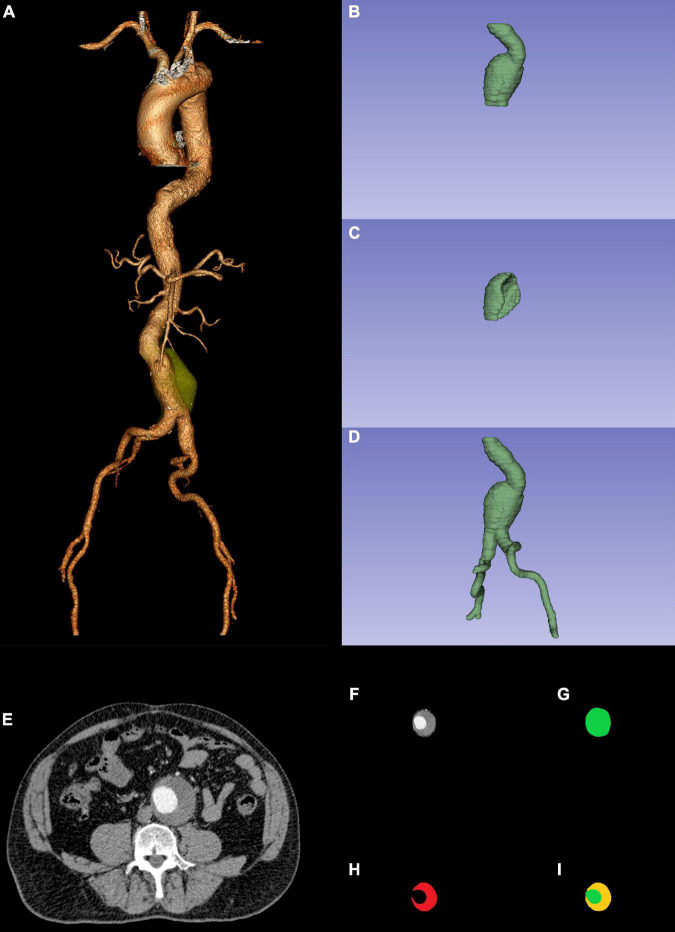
Preoperative CTA reconstruction (A); AAA sac ROI (B); ILT ROI (C); AAA ROI (D); AAA original ROI CTA slice (F) was the combination between AAA ROI CTA slicer (G) and original ROI CTA slice (E); CTA slicer (I) was the combination between AAA sac ROI CTA slicer (G) and ILT ROI CTA slicer (H). CTA, computed tomography angiography; AAA, abdominal aortic aneurysm; ROI, region of interest; ILT, intraluminal thrombosis.
